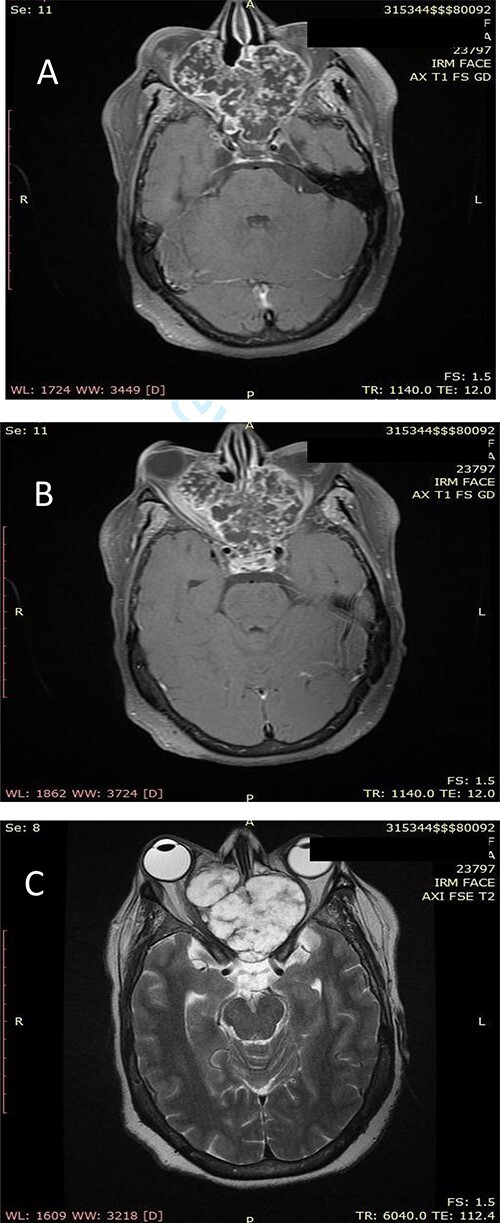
(A) Axial facial MRI in T1 sequence after Gadolinium injection showing a lobulated tissue process, occupying the nasal cavities, enhancing heterogeneous. (B) Axial facial MRI in T1 sequence after Gadolinium injection showing bilateral exophthalmos and a mass effect on the optic nerves. (C) Axial facial MRI in T2 sequence showing endocranial extension opposite the frontal lobes.
